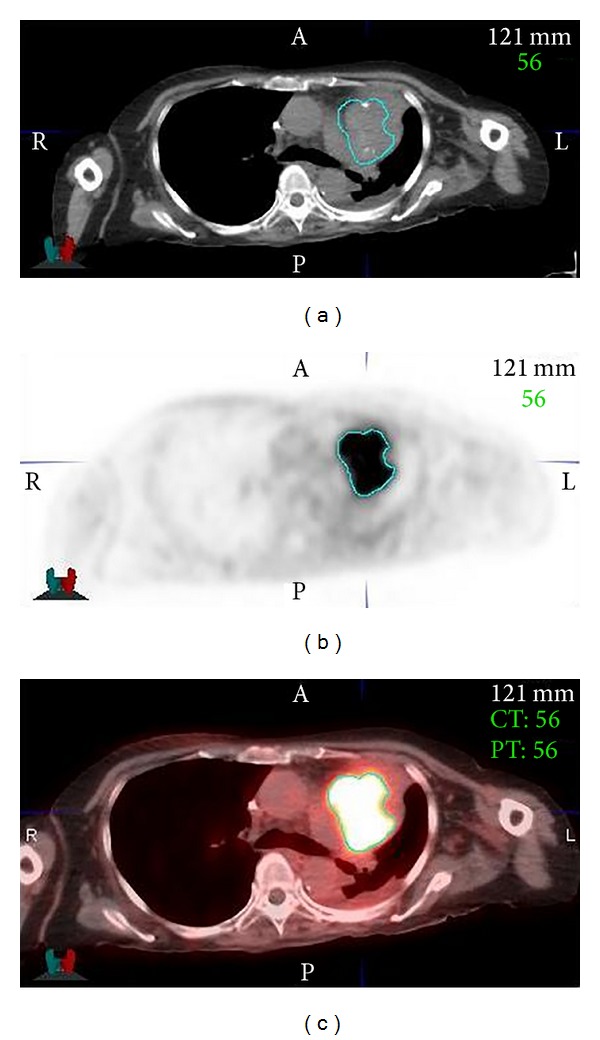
PET provides the ability to differentiate areas of neoplastic, hyper-metabolic activity within surrounding normal tissue where (a) is the CT image, (b) is the PET, and (c) is the fused PET/CT. All of the images show the gross tumor delineated from the PET image in teal color.
